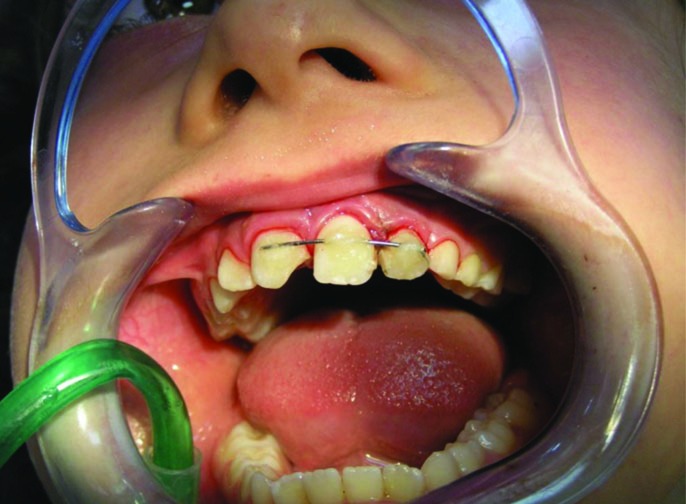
The splint was retained for 12 weeks. Radiographic and clinical examinations (sensitivity and percussion tests) were performed each month following repositioning. At the first two recall visits, the tooth did not respond to electrical and cold tests. However, the tooth was not tender to percussion and radiographic examination did not reveal any signs of developing pulp necrosis. Therefore endodontic intervention was not initiated. At the end of the third month the splint was removed (Figure 5) and the patient was scheduled for follow-up visits at 3-month intervals. It should be pointed out that during all the appointments, the vitality of other anterior maxillary and mandibular teeth was evaluated periodically.
